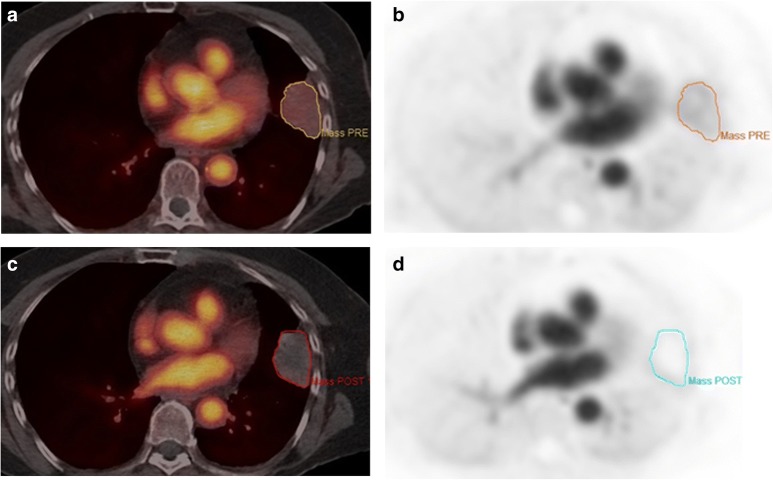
Baseline [^18^F]fluciclatide-axial fused PET/CT image (a) and corresponding PET image (b) with increased tracer uptake in left upper lobe pulmonary metastasis. Post 1 week of therapy while the lesion is still visualized on [^18^F]fluciclatide-PET/CT image (c), the corresponding PET image (d) shows a complete response to therapy
